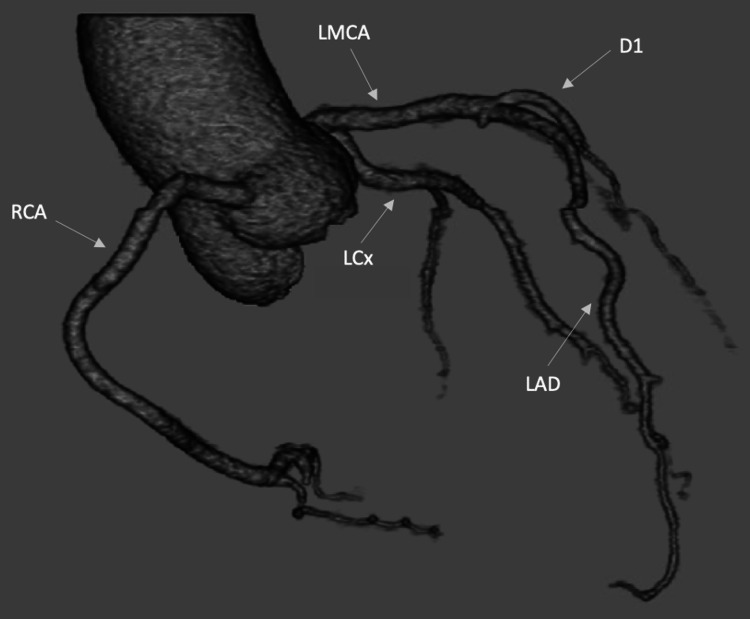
Three-dimensional reconstruction of coronary CT scan with no evidence of coronary disease RCA: right coronary artery; LMCA: left main coronary artery; LCx: left circumflex artery; LAD: left anterior descending artery; D1: diagonal artery.
